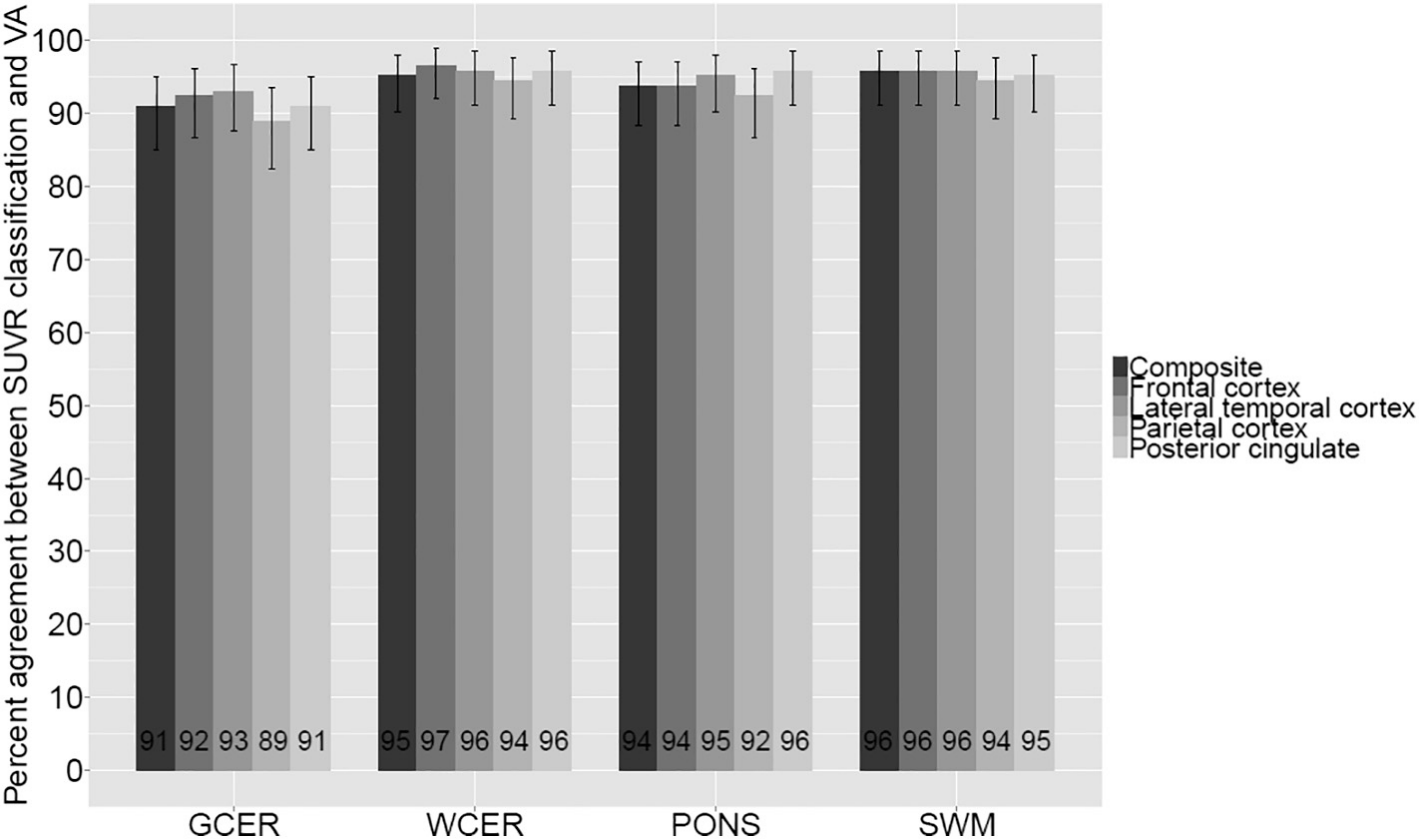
Percentage of agreement (and 95% confidence interval) between different SUVR cutoff classifications and VA in cohort A.
